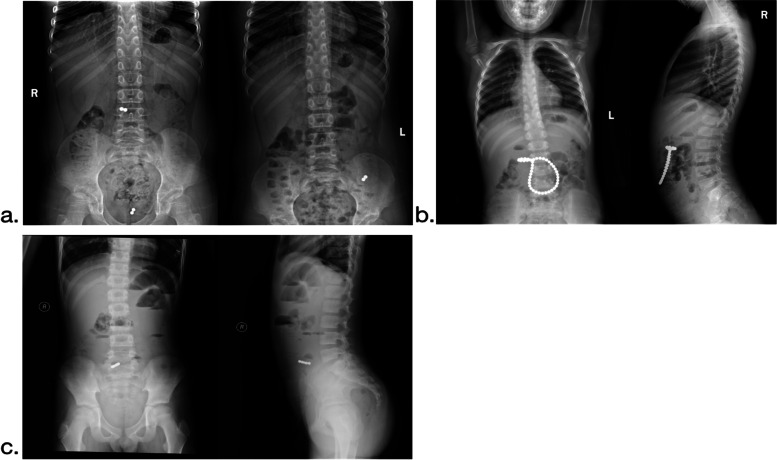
a 24-h dynamic radiographs for moving patients, and conservative treatment is performed. b The combined thoracic and abdominal radiograph confirms that the foreign body is in the duodenum, and the foreign body is extracted via gastroscopy. c During the operation, the combined chest-abdominal radiograph confirms the presence of magnetic foreign bodies causing intestinal obstruction. Meckel’s diverticulum is the location of the perforation in the digestive tract
